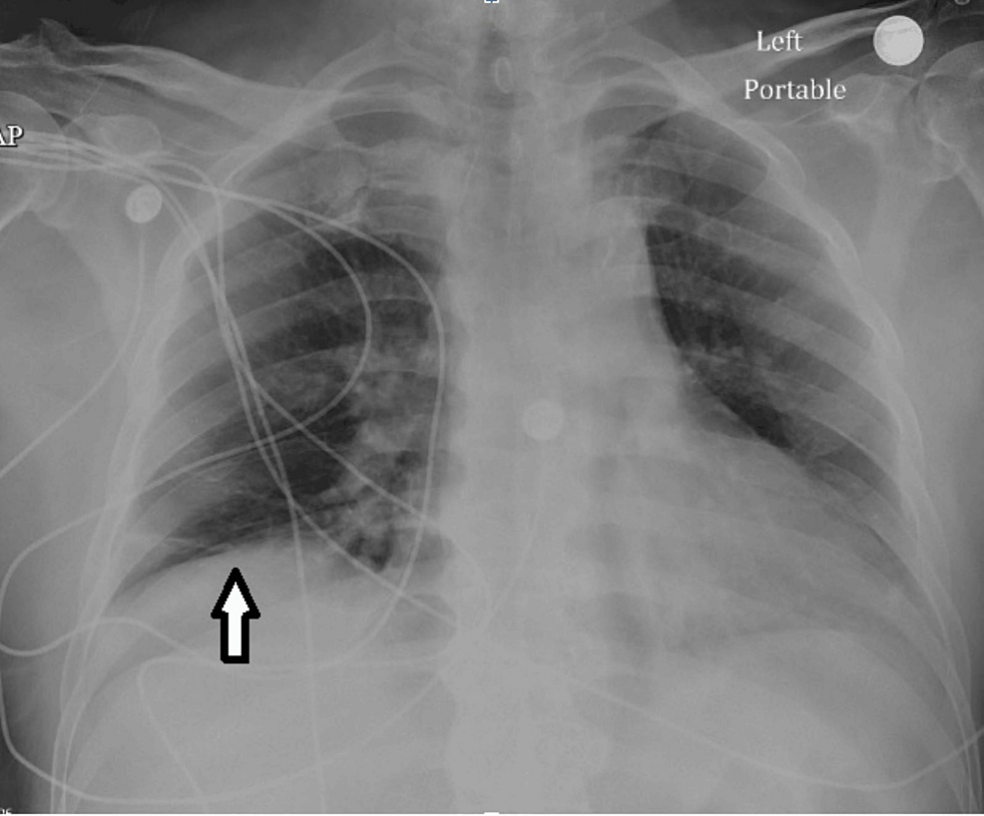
Chest X-ray on admission showing right basilar discoid atelectasis (white arrow).
